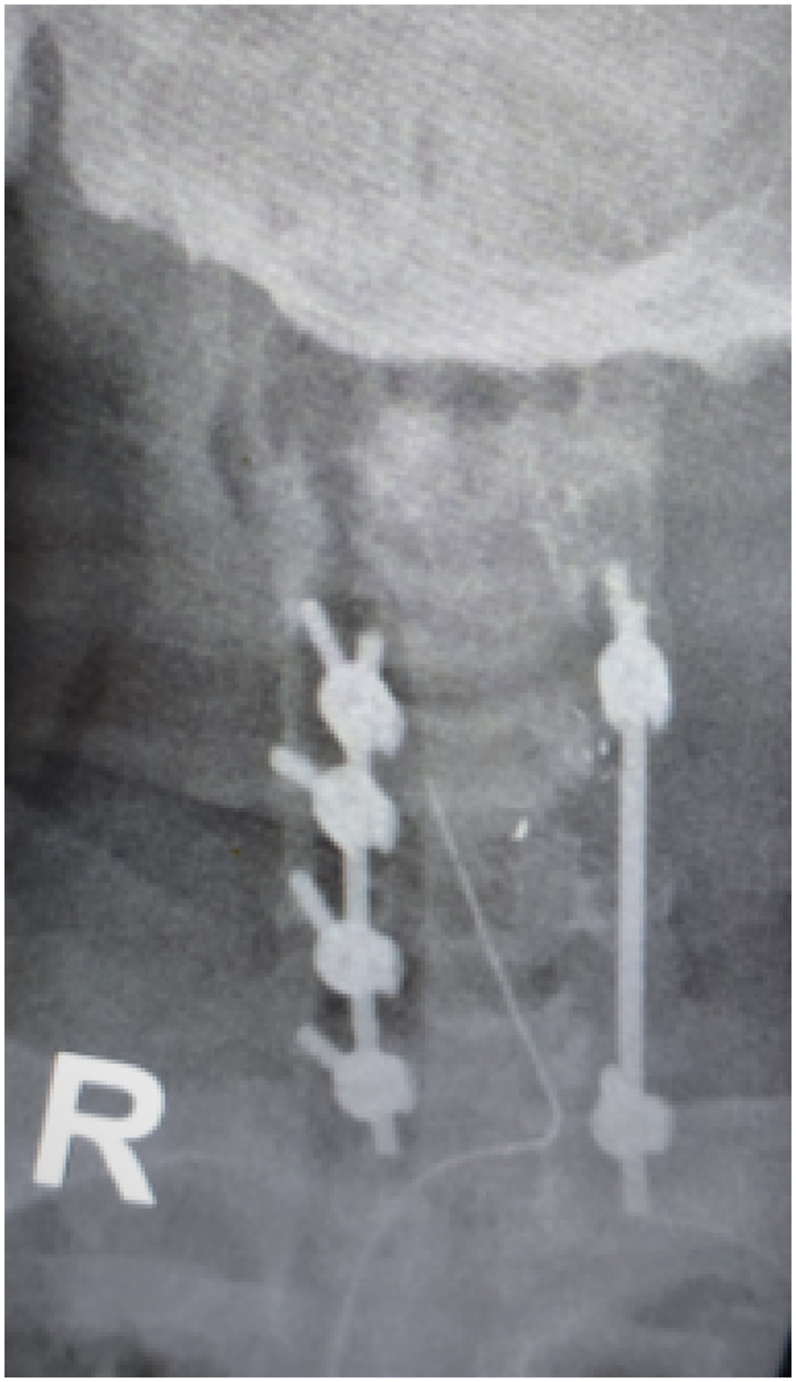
Post-operative plain cervical radiograph.
